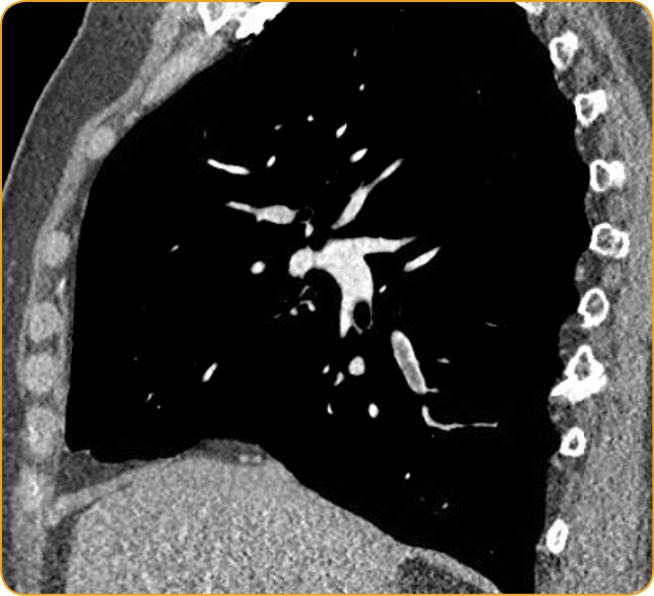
CT scan shows pulmonary embolism.
